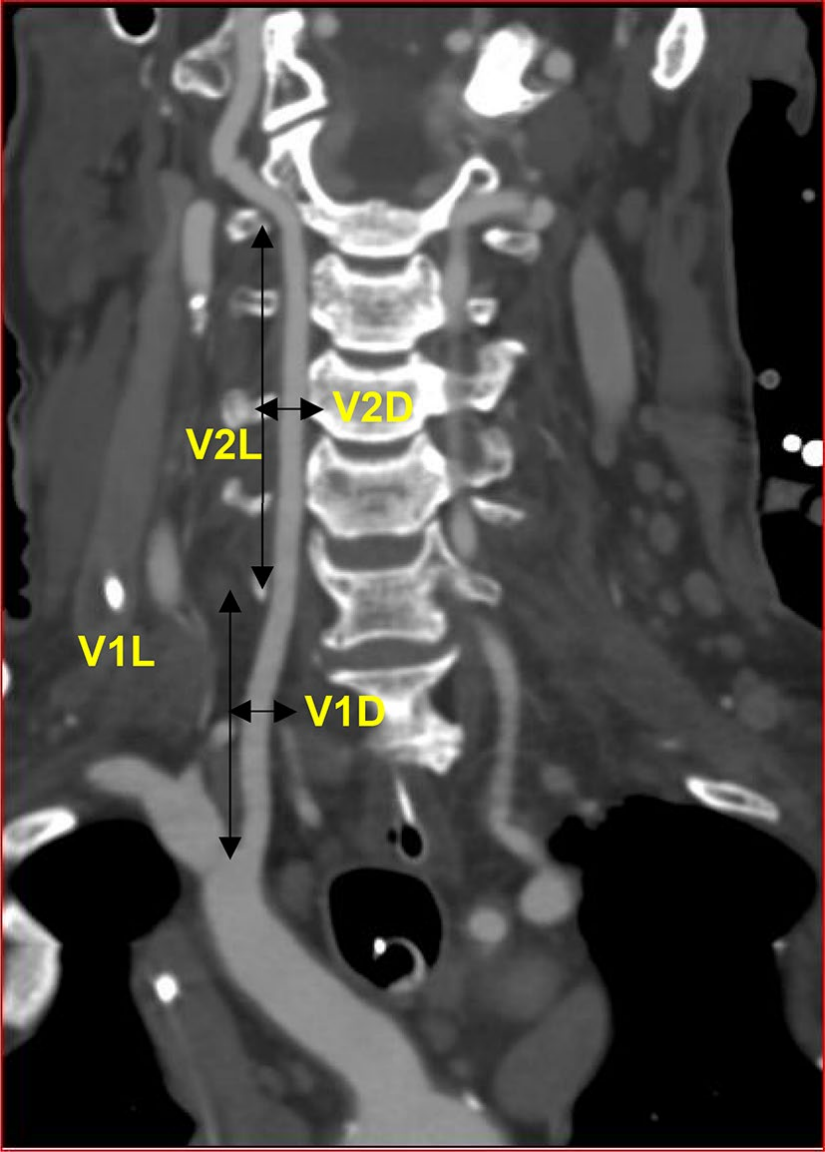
Coronal view of CTA image, V1 and V2 segments of the vertebral artery. Measured variables; V1L—the length of the VA from the origin to the point of entry into the transverse foramen; V2L—the length of the VA from the point of entry into the transverse foramen to the transverse foramen of the C2 cervical vertebra; V1D—the diameter of the V1 segment at a midpoint between origin and point of entry into the transverse foramen; V2D—the diameter of the VA V2 segment at a fixed point between C4 and C3 pedicles
